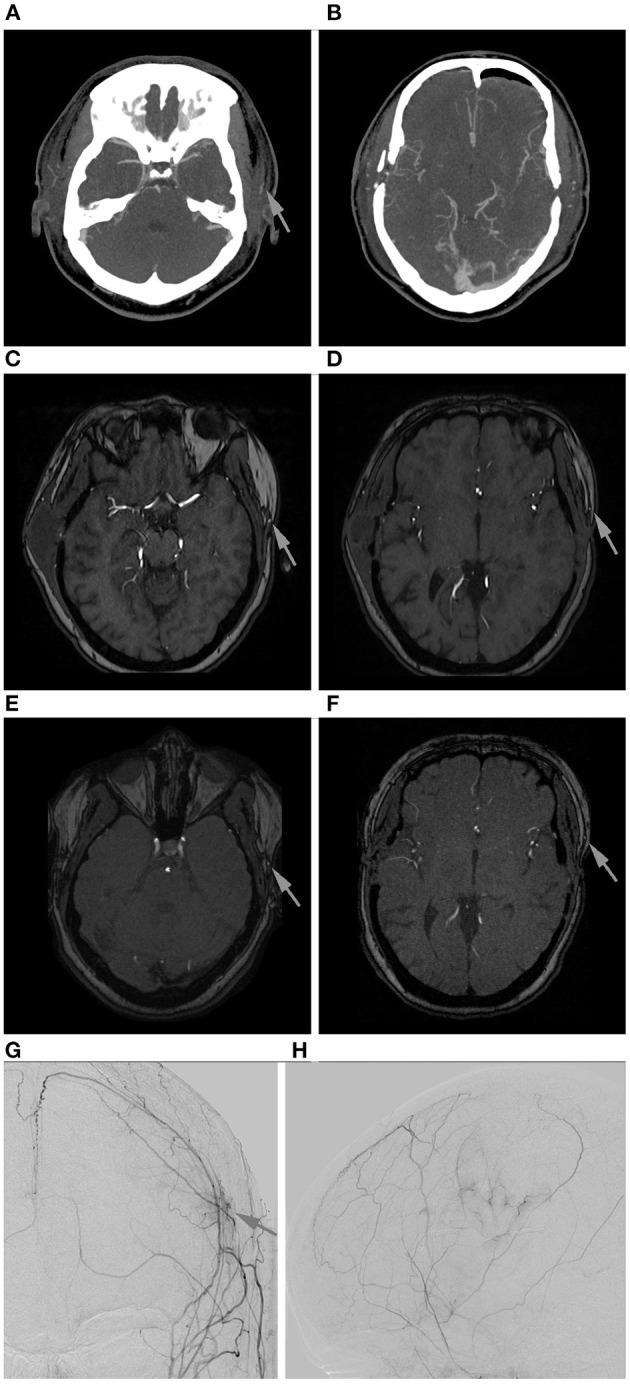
Follow-up of a left-sided STA-MCA bypass shown to be occluded in DSA. (A,B): Early post-operative CTA. Only the frontal branch of the STA is contrasted (arrow). (C,D): MR-TOF after 3 months. Only the frontal branch of the STA is contrasted (arrows). (E,F): MR-TOF after 6 months. Only the frontal branch of the STA is contrasted (arrows). (G,H): Angiogram of the external carotid artery anterior-posterior (G) and lateral (H) with only little cortical perfusion at the site of revascularization (arrow; vessel sprouting caused by indirect revascularization with additional EGPS (MCA territory) and in the ACA territory (spontaneous collateralization caused by the middle meningeal artery).
